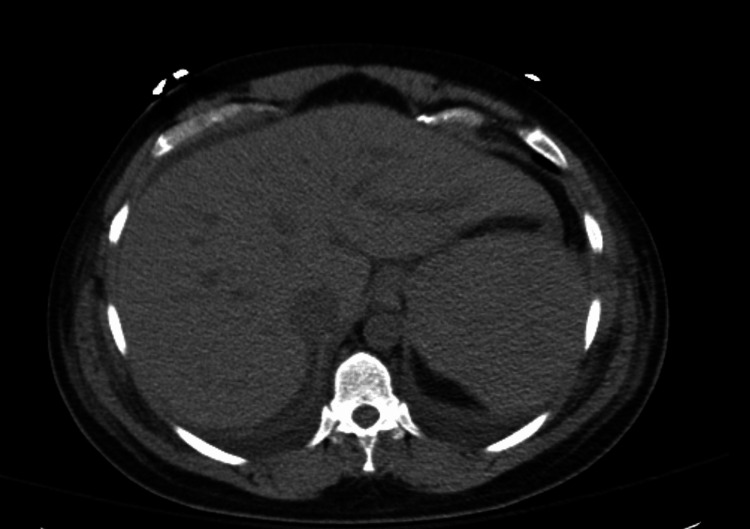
CT scan showing hepatomegaly with mild ascites.
